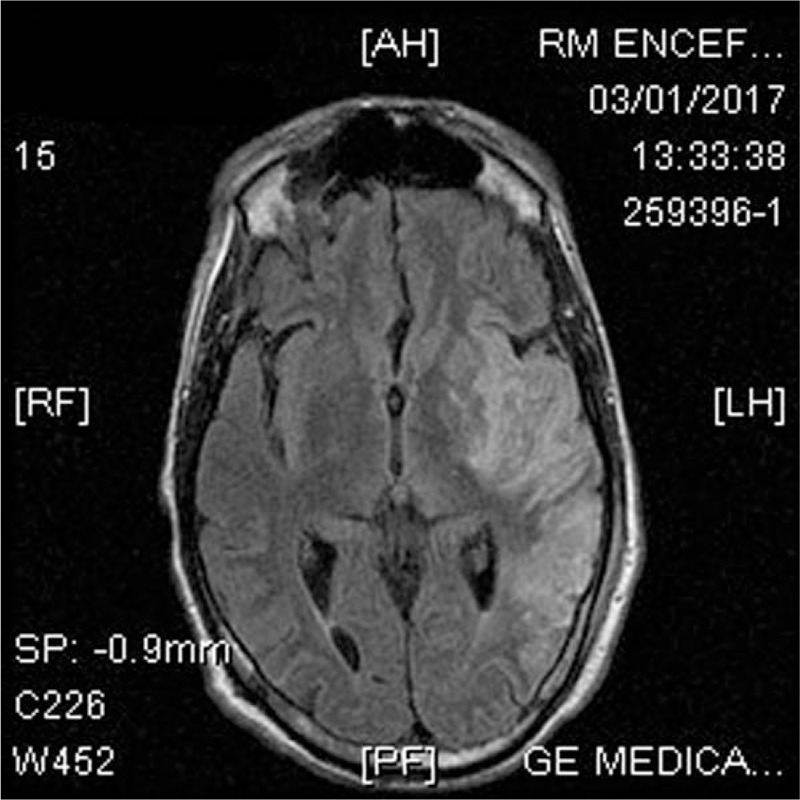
Brain MRI shows an acute infarction in the left hemisphere.
